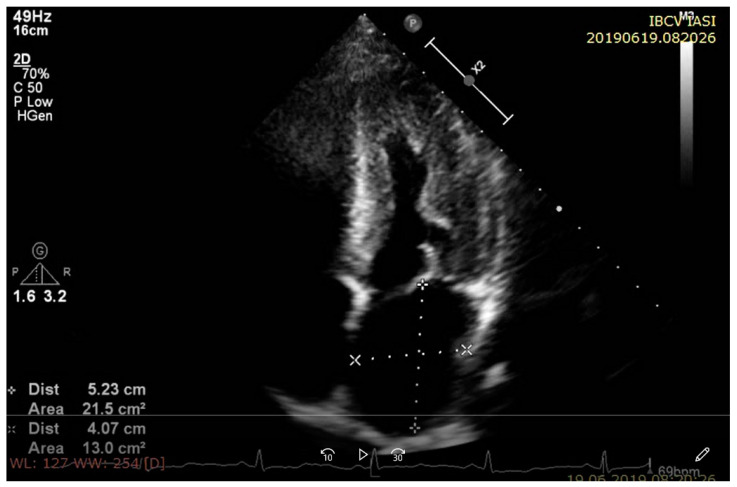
Transthoracic echocardiogram, apical four-chamber view in a patient with cardiac amyloidosis showing: increased thickness of left ventricle wall with “granular sparkling” appearance, increased biatrial dimensions in contrast with a small left ventricle cavity, and pericardial effusion.
